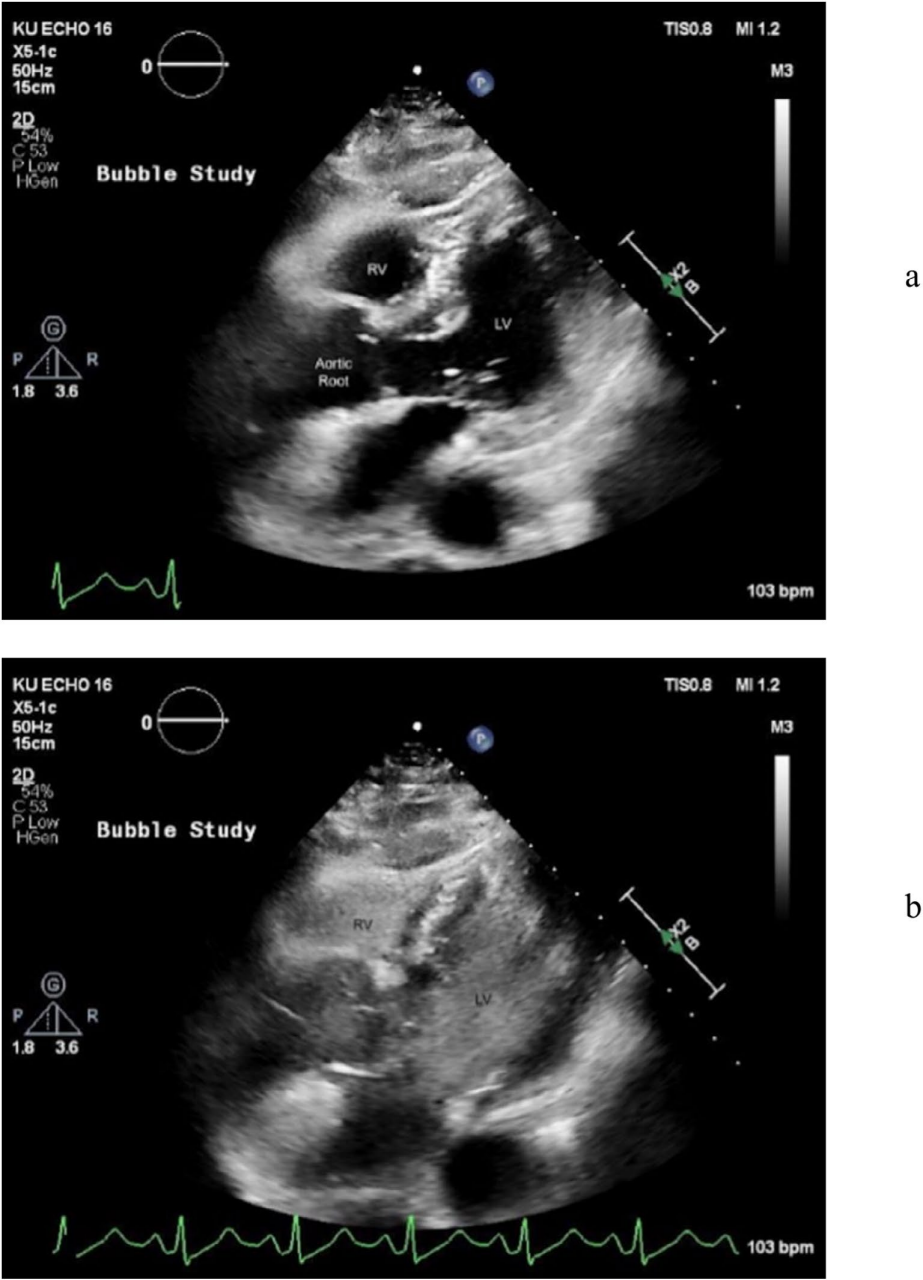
Transthoracic echocardiogram images of pre‐ (a) and post‐ (b) bubble study demonstrating complete and equal opacification of both the right ventricle (RV) and the left ventricle (LV) that make the blood appear speckled white and gray.
